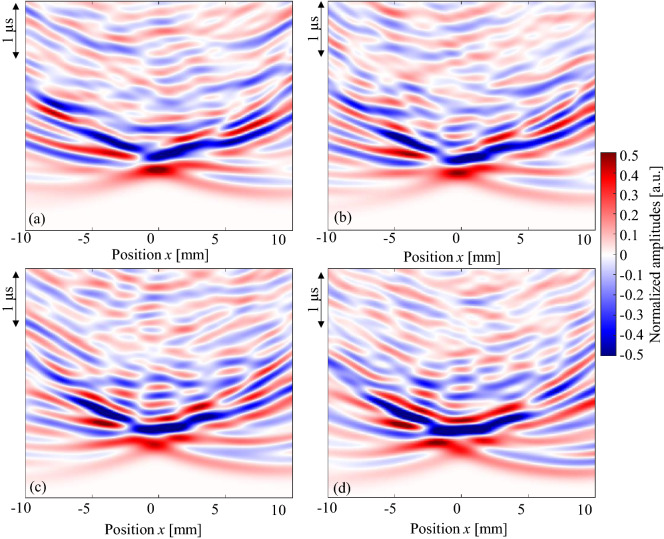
Observed waves at different positions. Ultrasonic waves propagated through models of sample A with different BV/TV values; (a) 56%, (b) 62%, (c) 68% and (d) 73%.
